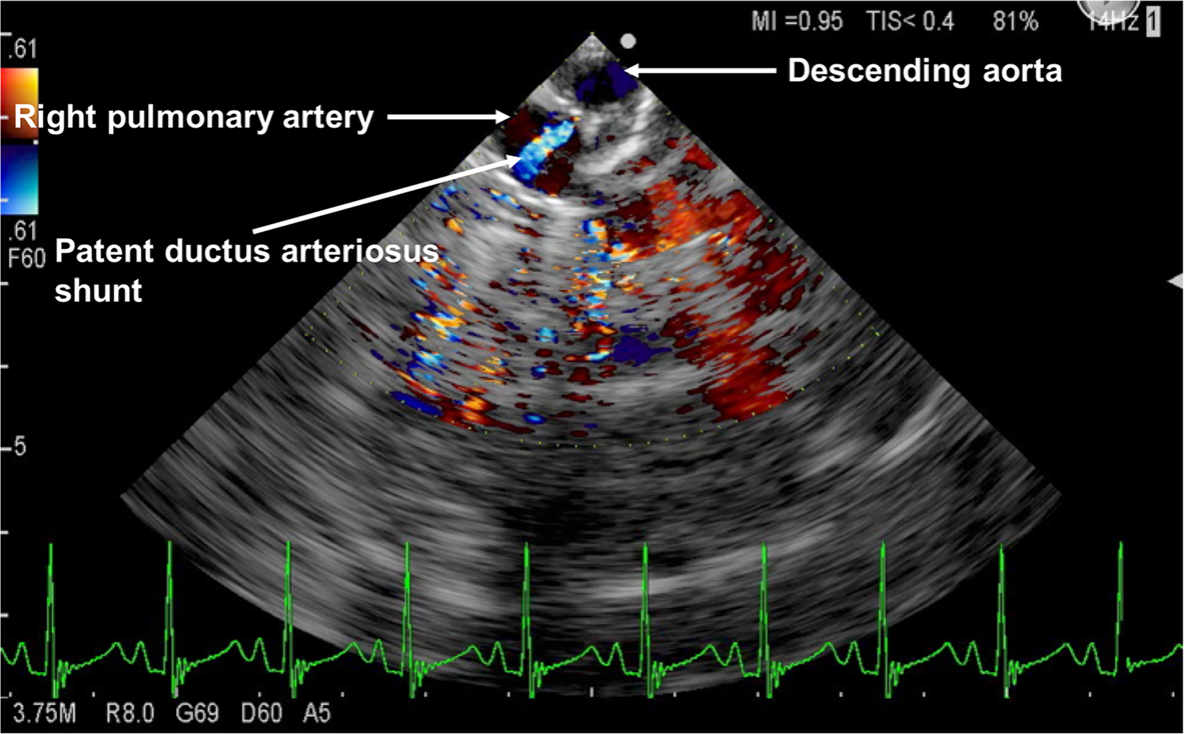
Residual PDA shunt flow on color Doppler flow imaging. A mosaic jet moving from the descending aorta toward the pulmonary artery, indicating a residual PDA shunt, is seen
